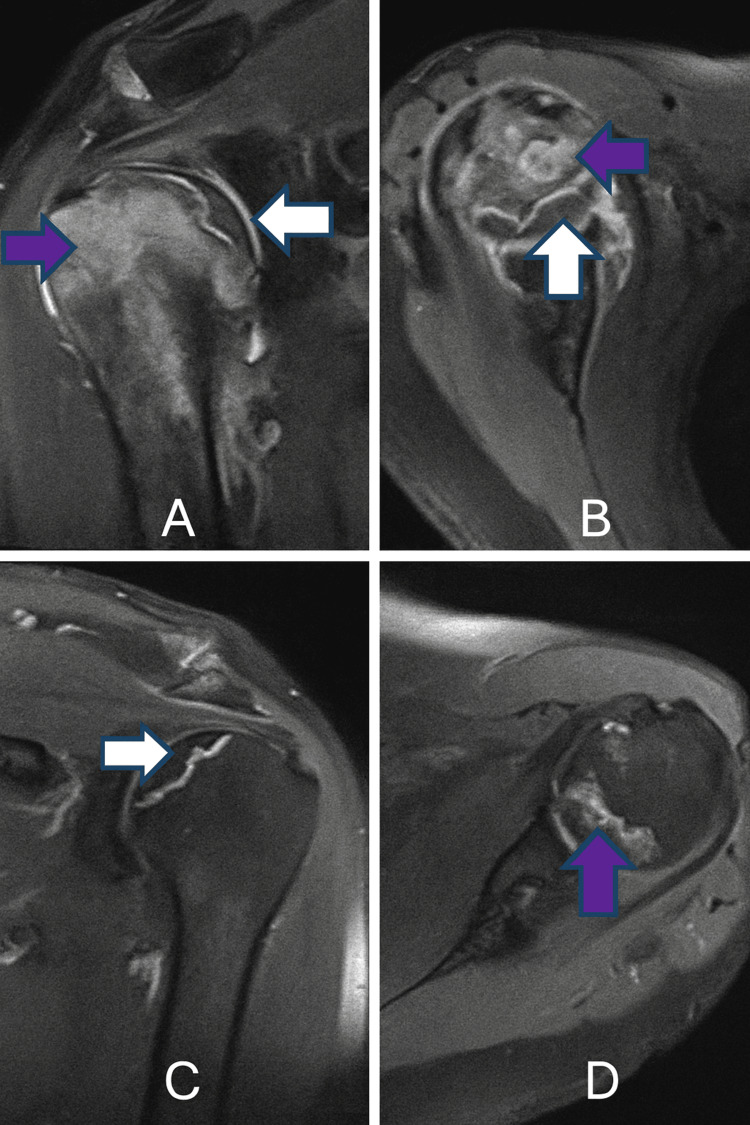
Magnetic resonance imaging (MRI) findings of bilateral shoulder osteonecrosis (A) Coronal MRI of the right shoulder showing a crescentic subchondral lesion (white arrow) and necrotic bone marrow (purple arrow).
